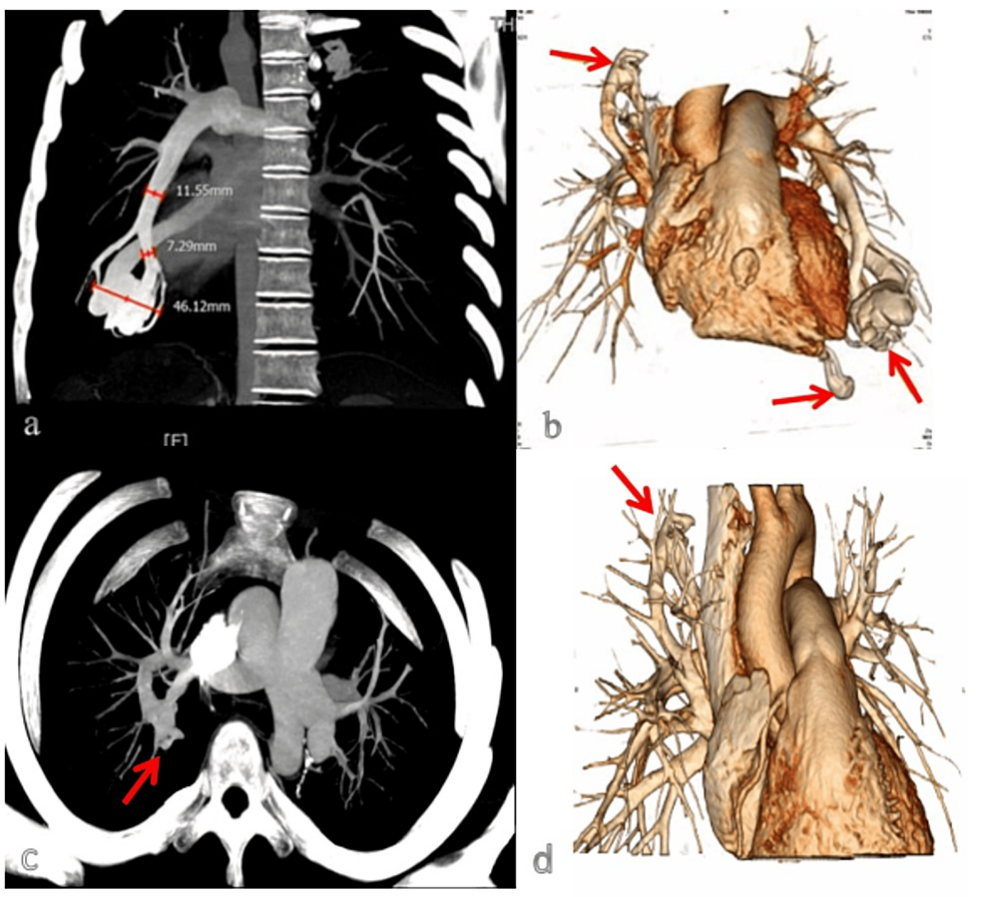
(a) The pulmonary arteriovenous fistula drainage artery diameter was approximately 11.55 mm. (b) The pulmonary artery 3D-CT before the operation. The pulmonary angiography (c) and pulmonary artery 3D-CT (d) at six months after the operation.
